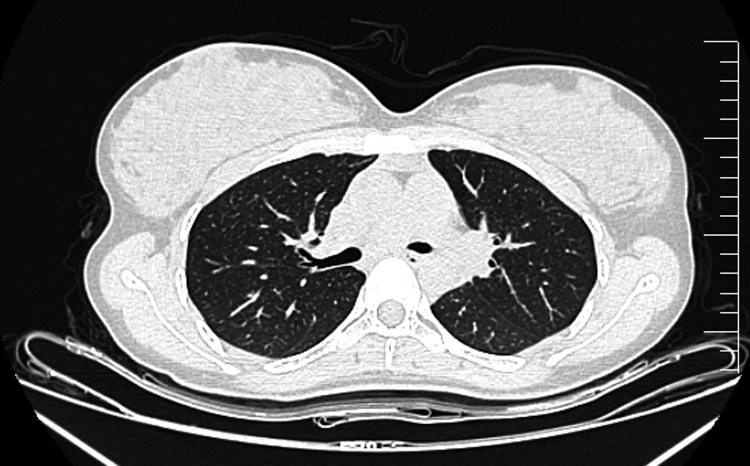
High-resolution computed tomography (CT) scan of bilateral lung fields showing no pathological lesion and no lymphadenopathy.
